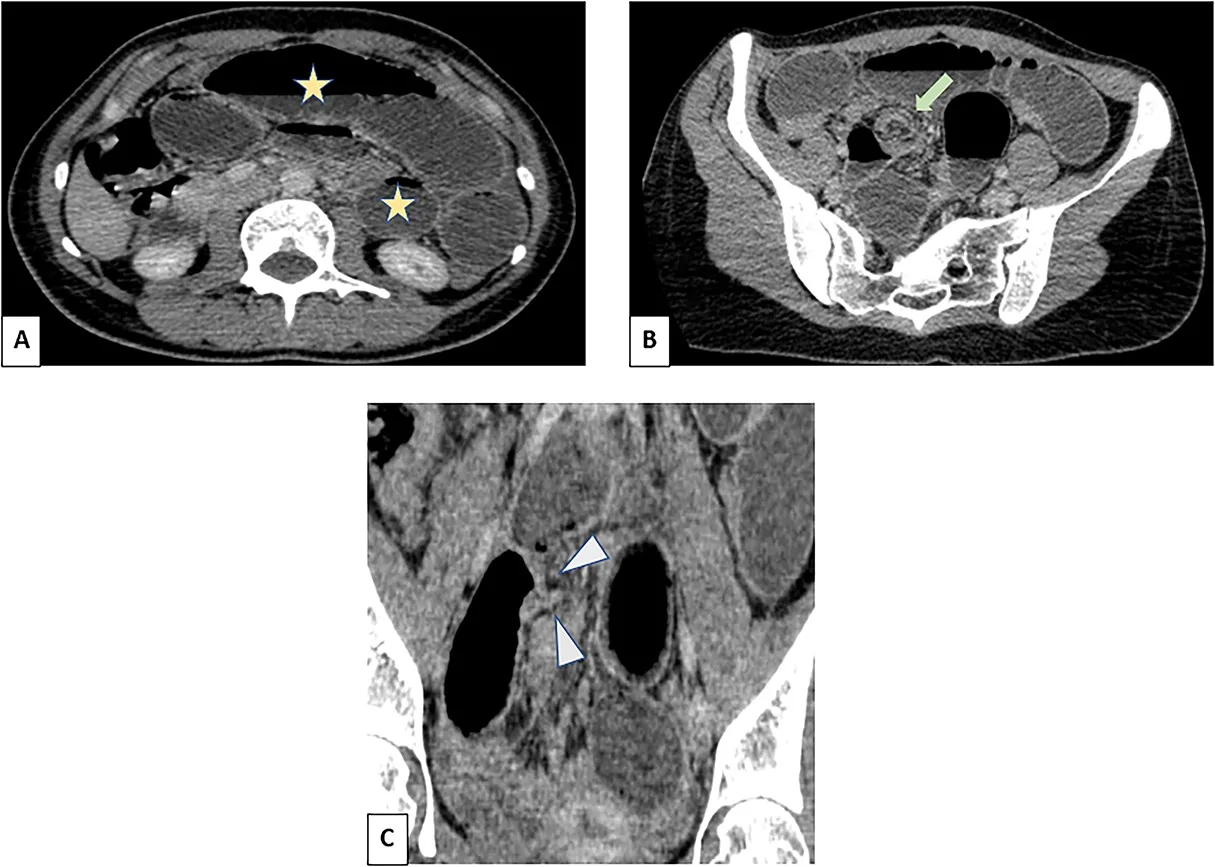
Contrast-enhanced abdominal CT showing dilated small-bowel loops with air–fluid levels (asterisks) proximal to a focal transition point forming the beak sign (arrow). Twisting of the bowel loops and mesenteric vessels produces the whirl sign (arrowhead). At the same level, a focal extraluminal fatty indentation corresponding to the fatty notch sign is identified, reflecting mesenteric fat insinuation at the transition point.
